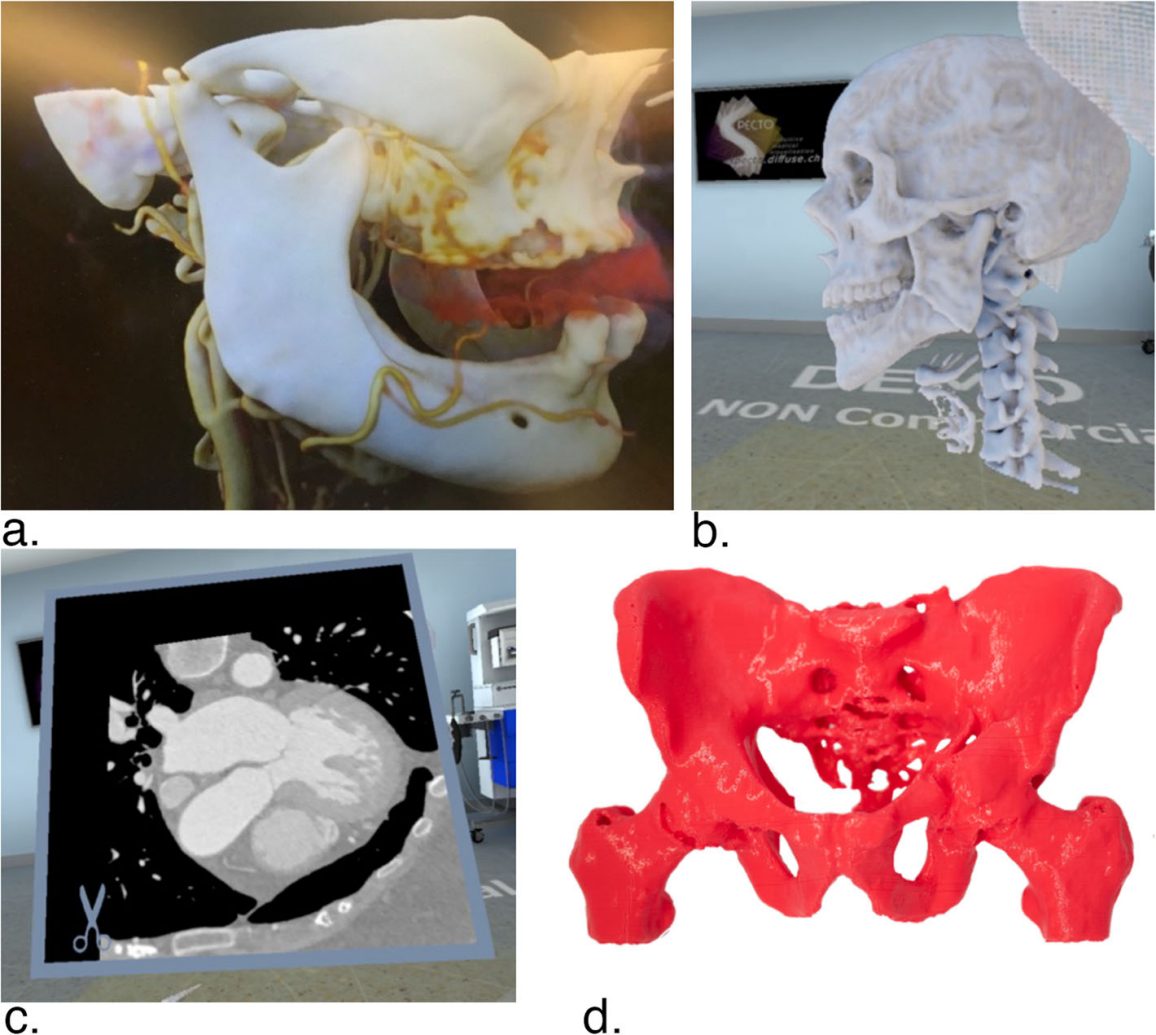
Examples of prepared cases.* a. Dislocated right condylar fracture of the mandible *on 3D-Display (picture taken through passive shutter glasses).* b. Angle mandibular fracture *on VR-Glasses (corresponding two-dimensional view on computer-display).* c. Proximal crossing of RIVA and RCX *on VR-Glasses, CT View (corresponding two-dimensional view on computer-display).* d. Posterior column fracture left, 3D-printed. Data: RIVA = Ramus interventricularis anterior, RCX = Ramus circumflexus
